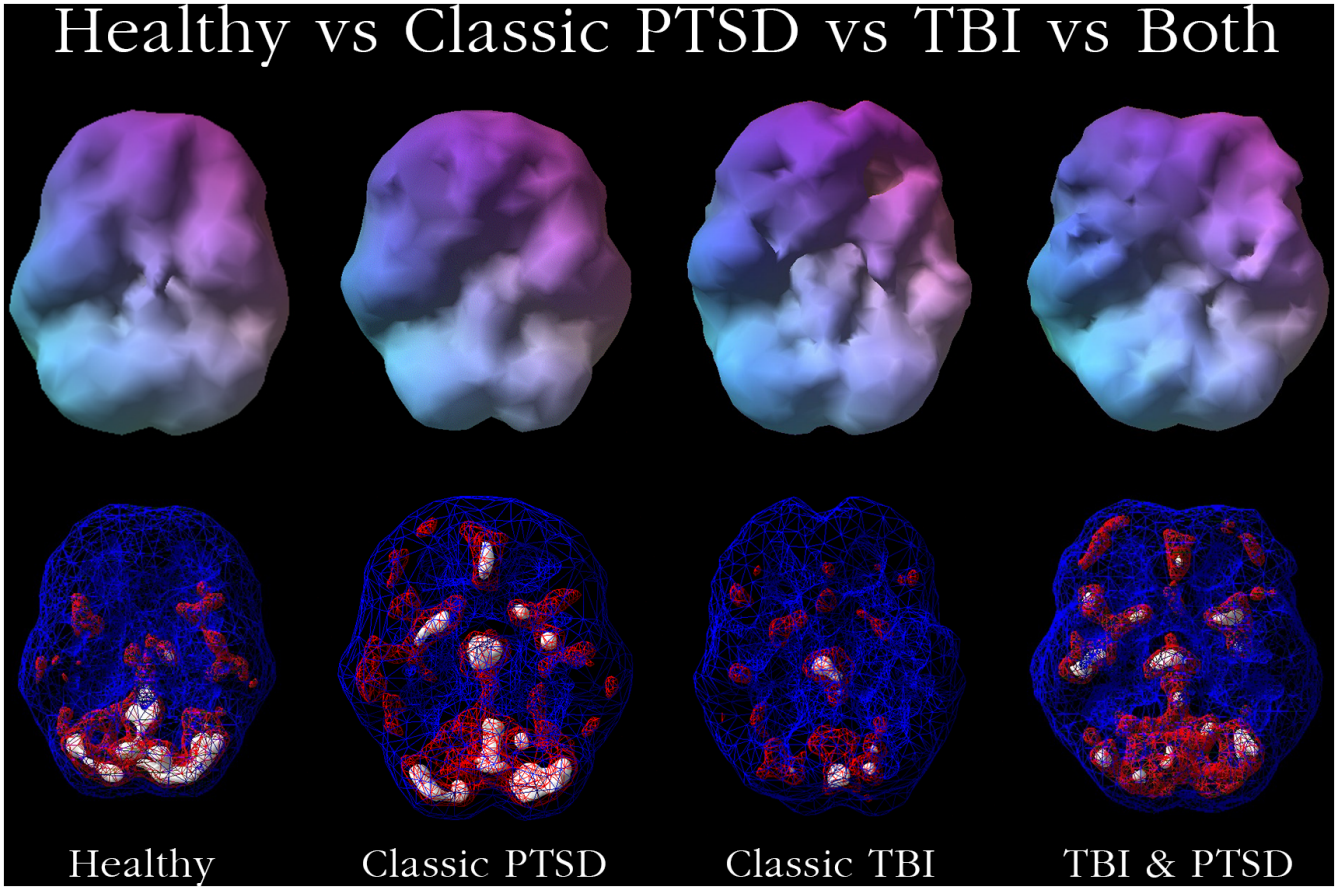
Brain SPECT Images of Healthy, PTSD, TBI and PTSD Co-morbid with TBI Perfusion Patterns. Top row, underneath surface scans, threshold set at 55%, looking at top 45% of brain perfusion. Bottom row, underneath active scans where blue = 55%, looking at top 45% of brain perfusion, red = 85% and white 93%. Healthy shows full even, symmetrical perfusion with most active area in cerebellum. Classic PTSD shows increased anterior cingulate, basal ganglia and thalamus perfusion. Classic TBI shows multiple areas of low perfusion seen on surface scans (top row). TBI and PTSD show both.
